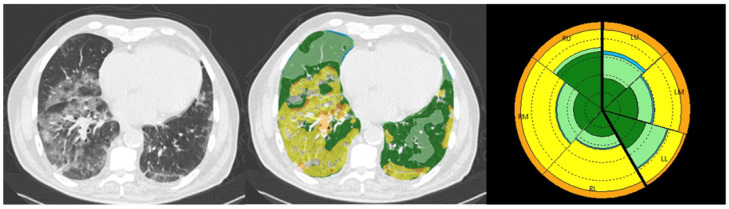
CALIPER analysis of a CT scan in a 73-year-old male patient. From left to right: axial CT slice, axial CALIPER-derived color image overlays (dark and light green = norm, yellow = GG, orange = Ret), the glyph of the lung parenchymal patterns.
